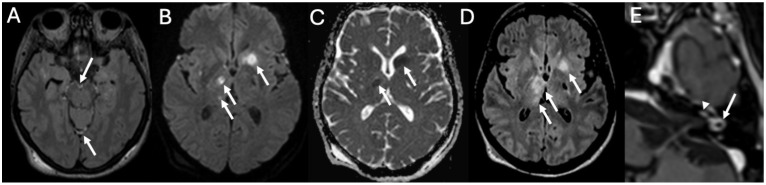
Inner ear involvement in a 64-year-old patient with Pneumococcal Meningitis. Axial FLAIR (A), DWI (B), ADC (C), enhanced FLAIR (D), and T1 (E) show leptomeningeal enhancement (arrows in (A)), multiple ischemic areas (arrows in (B–D)) and enhancement of the basal turn of the right cochlea (arrowhead in (E)) and ipsilateral lateral semicircular canal (arrow in (E)) due to labyrinthitis.
